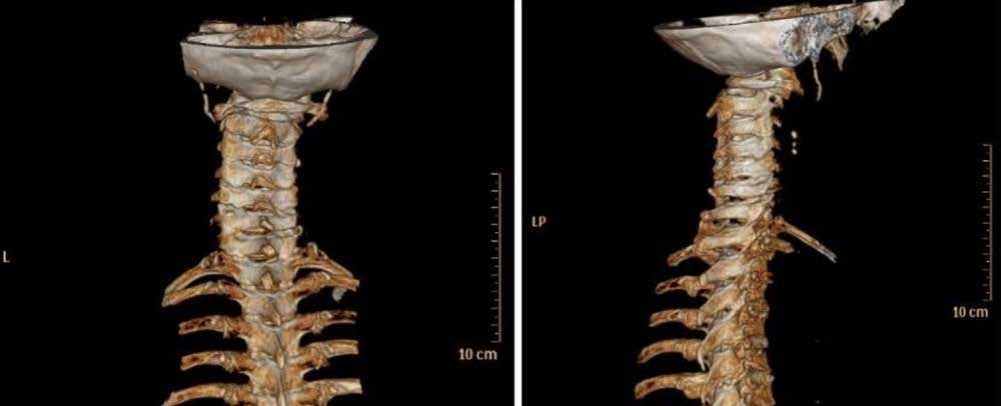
Cervical spine three-dimensional CT: Atlantodontic joint and C1/2 bilateral vertebral surface hyperplasia and sclerosis, local bone resorption and soft tissue shadow under the left vertebral surface of C1/2, corresponding horizontal spinal canal stenosis, atlantoaxial joint subluxation. CT = computed tomography.
